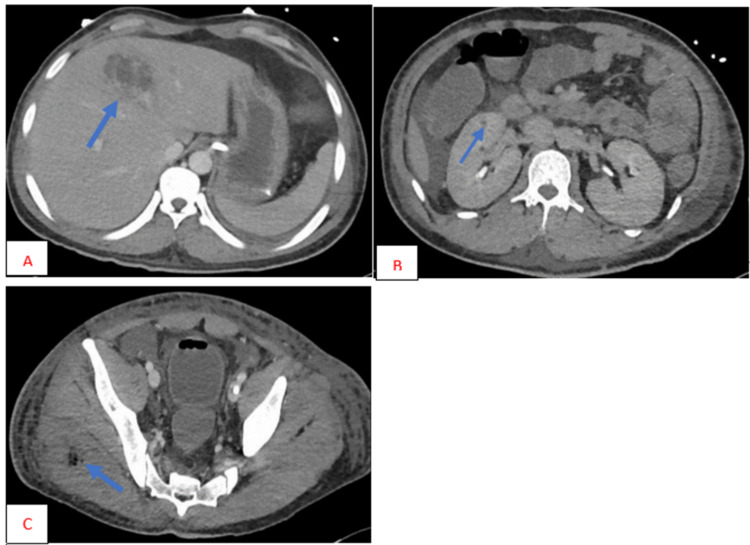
CT of the abdomen The images showing (A) liver abscess, (B) kidney abscess, and (C) gluteal muscle abscess (blue arrows) CT: computed tomography
